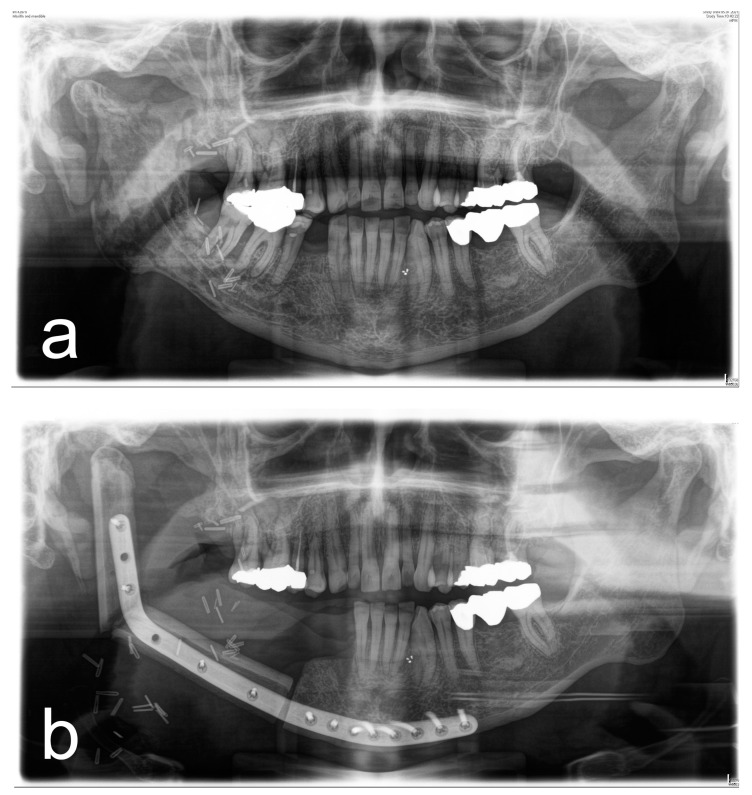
(a) Panoramic X-ray of a patient with extensive ORN of the mandible. (b) Postoperative panoramic X-ray of the same patient after ablative and reconstructive surgical therapy of ORN using a vascularized 2-segment fibula flap with intraoral soft-tissue transfer and patient-specific implant.
